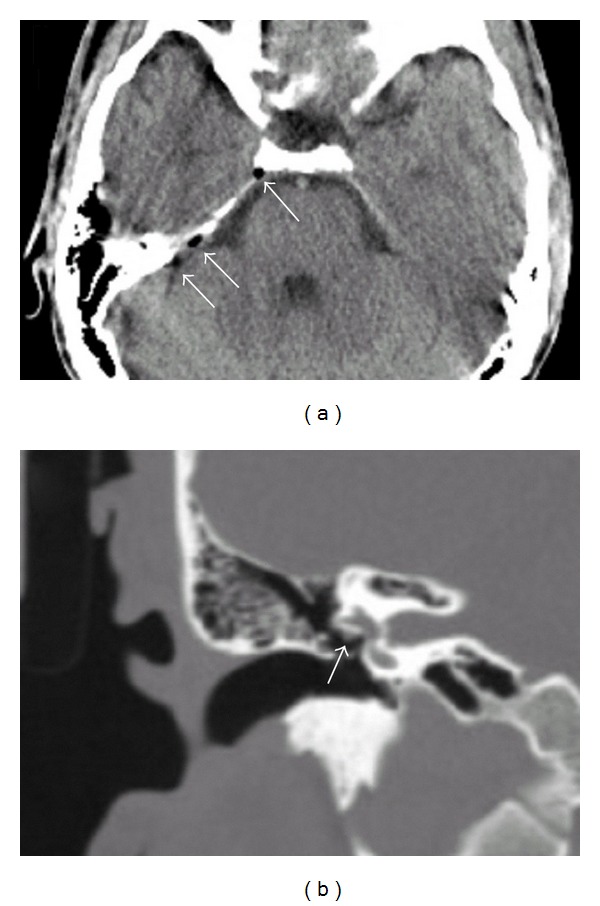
(a) Brain CT (axial section) free air (arrow) is observed in the subdural space. (b) Temporal bone target CT (sagittal section); the tympanic segment of the facial nerve (arrow) appears to be directly exposed to the tympanic cavity.
